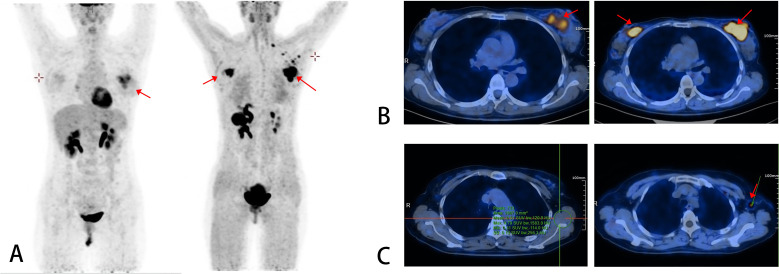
Comparison of two PET/CT images before neoadjuvant therapy. In (A–C), the left images are [^18^F]FDG PET/CT scans, and the right images are [^18^F]AlF-NOTA-FAPI-04 PET/CT scans. The [^18^F]AlF-NOTA-FAPI-04 PET/CT scans demonstrate clear visualization of the primary lesions with high uptake on both sides, as well as the metastatic lesion in the left axillary lymph nodes.
